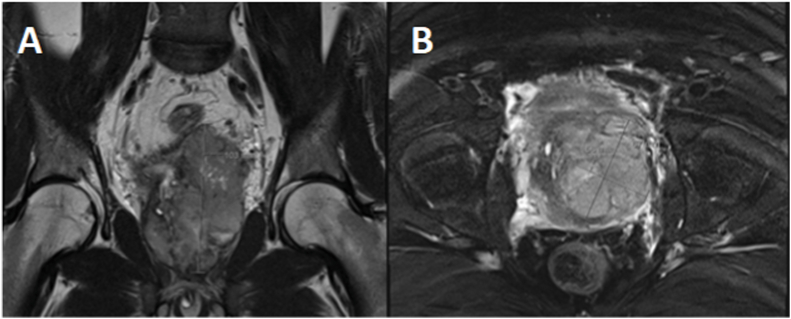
Panel A - Coronal MRI Imaging Following Transurethral Resection showing Irregular Prostatomegaly with Intravesical Protrusion and Internal Necrosis Panel B – Axial MRI Imaging Following Transurethral Resection showing Irregular Prostatomegaly with Intravesical Protrusion and Internal Necrosis.
